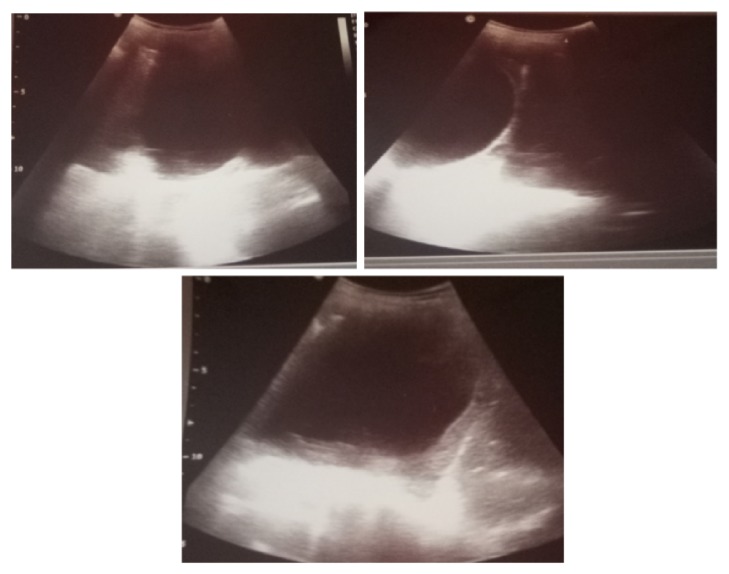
Thoracic ultrasound showing an intrapleural cyst with a proliferative membrane (patient 1).
